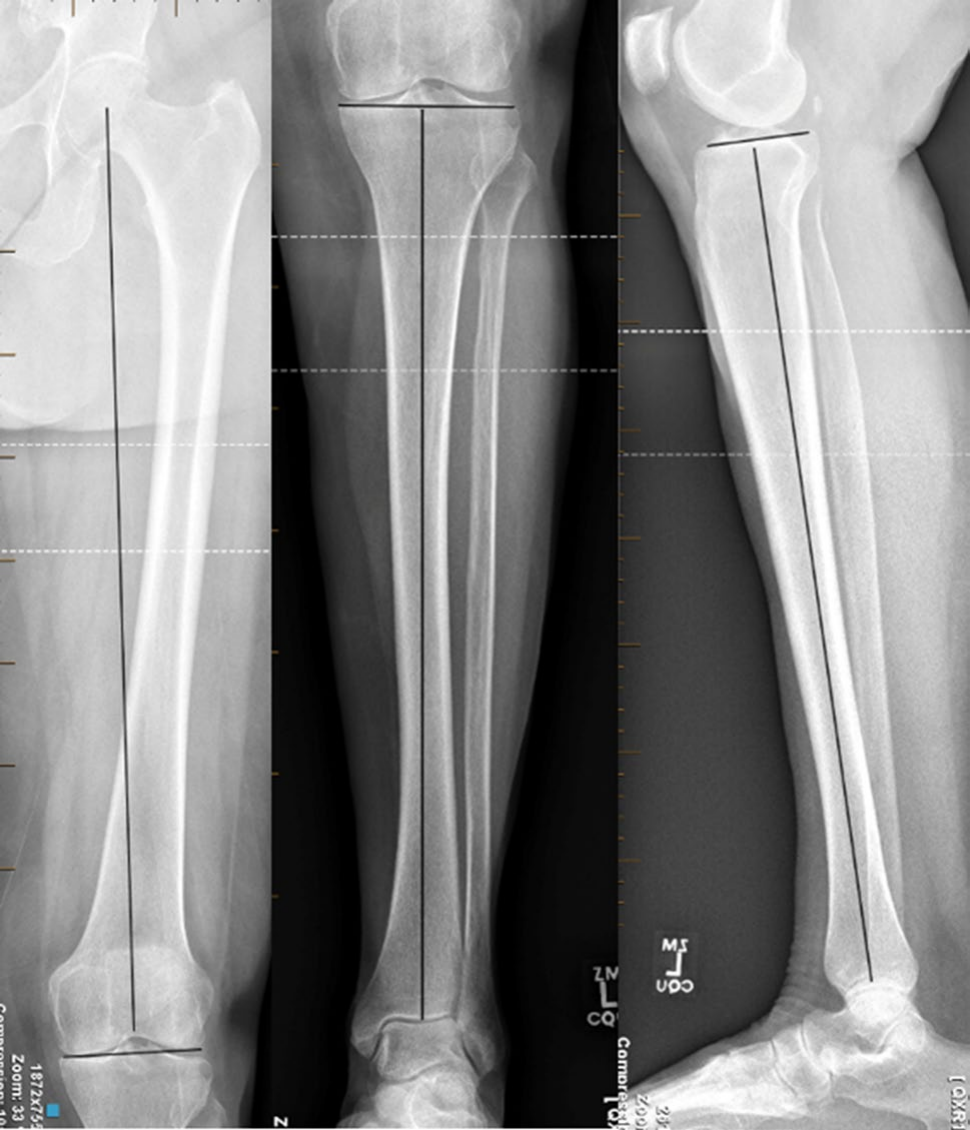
Radiographic parameters demonstrating measurement of the; mechanical Lateral Distal Femoral Angle (mLDFA), mechanical Proximal Tibial Angle (mPTA) and Posterior Tibial Slope (PTS)
